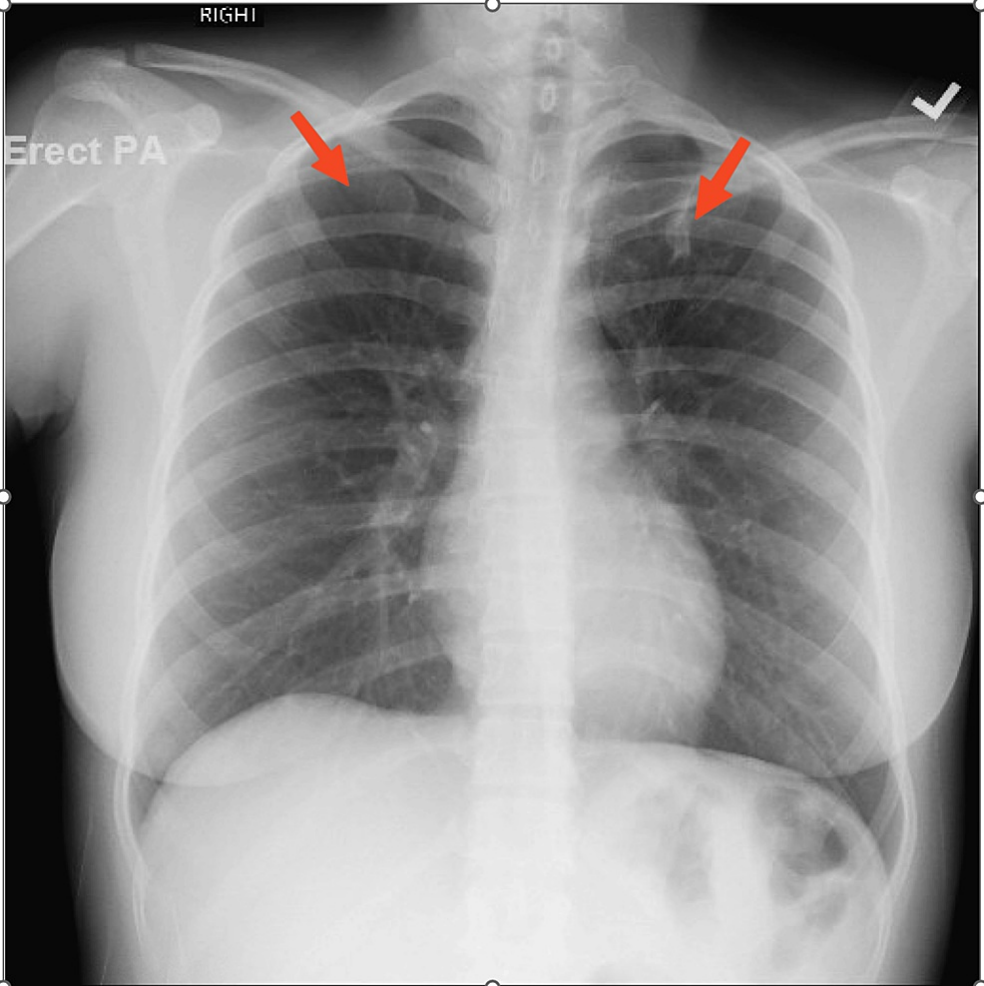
Chest X-ray demonstrating apical lobe scarring/atelectasis (red arrows).
